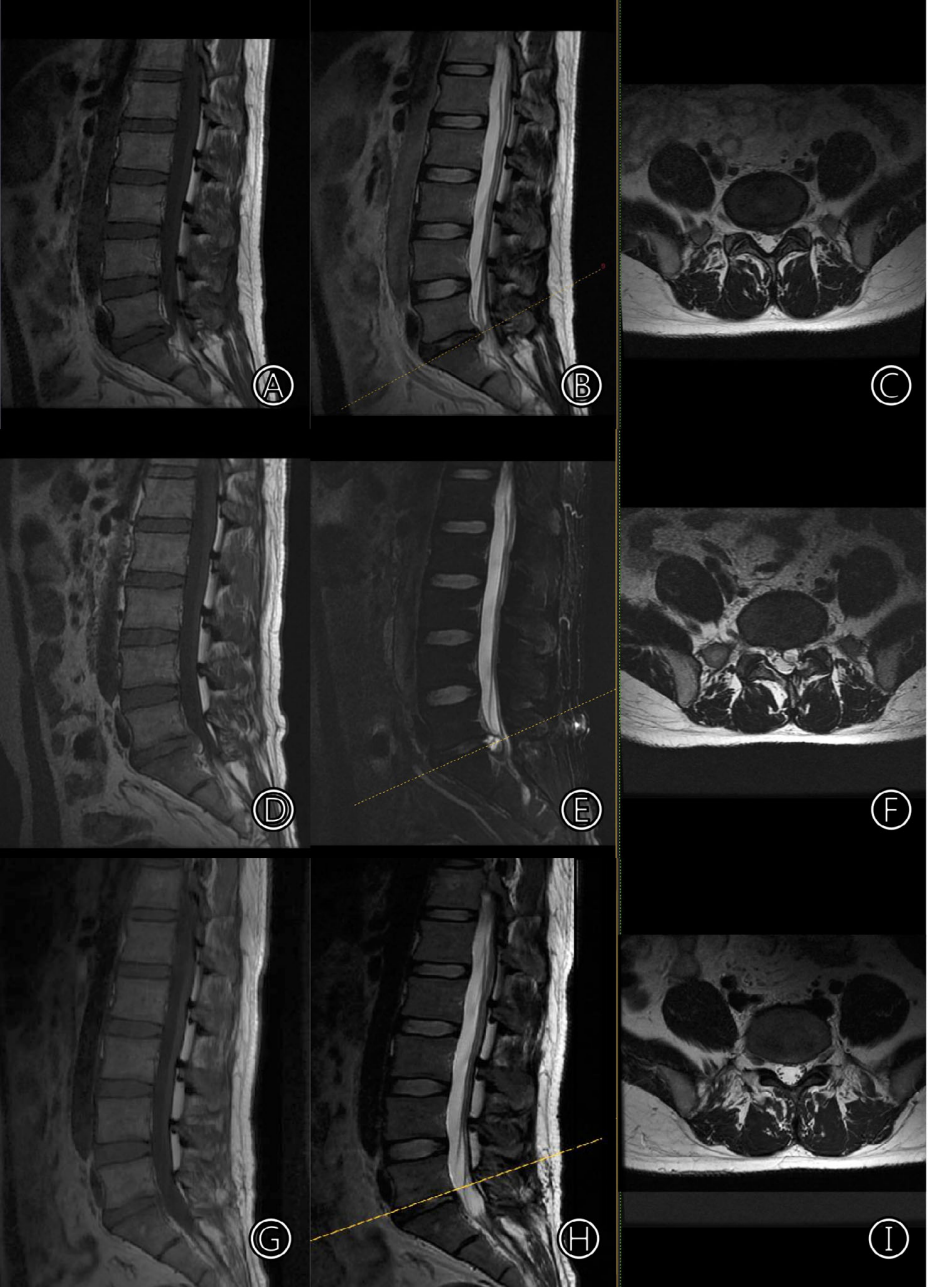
Preoperative MRI showed that the left part of the intervertebral disc of L_5_S_1_ protruded and compressed the nerve root of S_1_ (A–C). MRI on the 40th day after the first operation suggested a postoperative discal pseudocyst (PDP) (D–F). At the 6‐month follow‐up after the second operation, the MRI showed that the PDP had disappeared (G–I).
